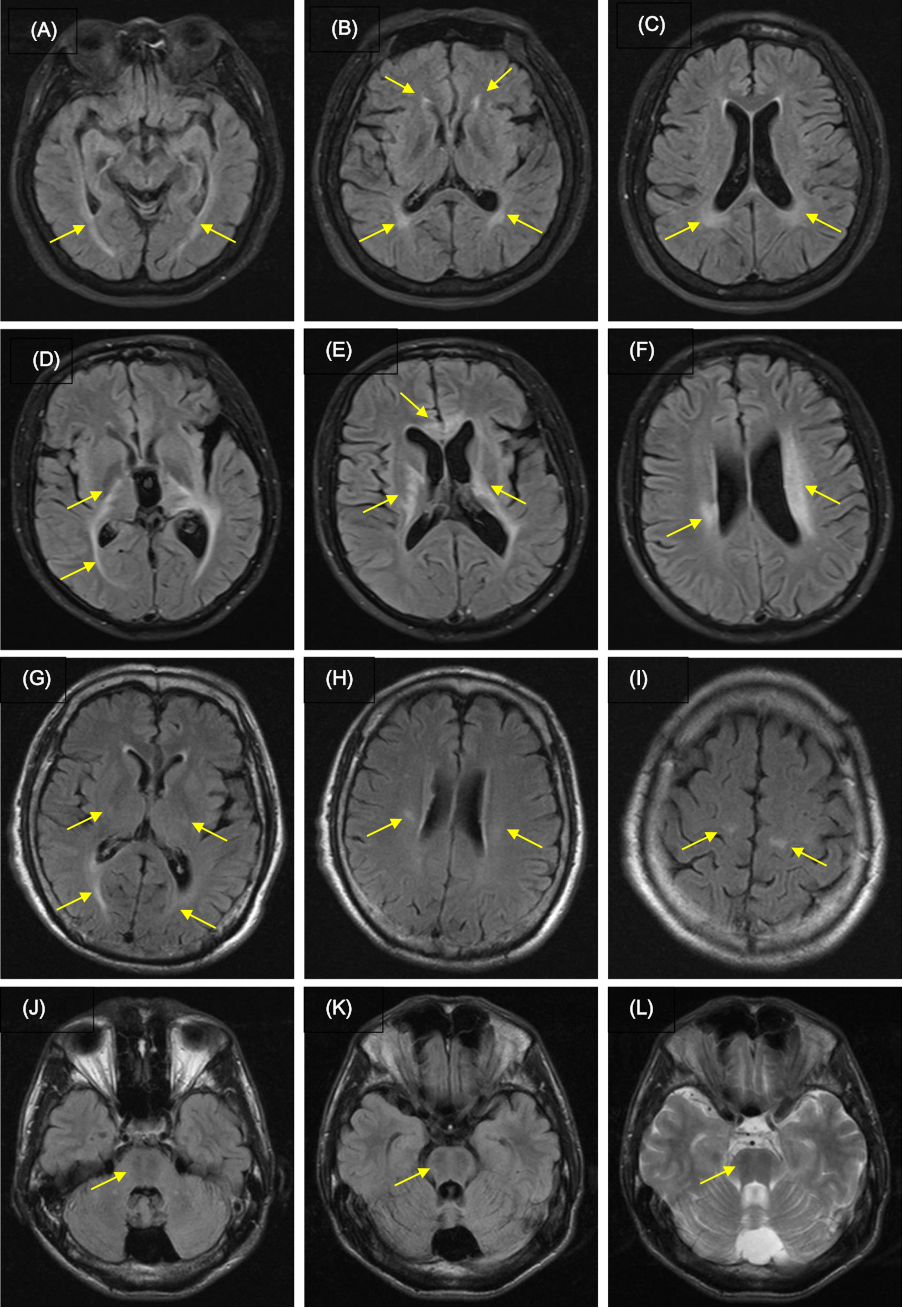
The brain MRIs of four cases. a case 14/SPG35, FLAIR sequences showed hyperintensities in the white matter (A-C) b case 22/X-ALD, FLAIR sequences demonstrated bilateral hyperintensities in the deep white matter, corpus callosum and internal capsules (D-F) c. case 25/Krabbe’s Disease, FLAIR sequences showed hyperintensities  in the corticospinal tracts starting in the precentral gyrus and extending to corona radiata, posterior limb of internal capsules, as well as in the terminal areas of white matter (G-I) d. case 27/ARSACS, T2-FLAIR-sequences showed linear hypointensity in the pons and cerebellar atrophy (J-L)
